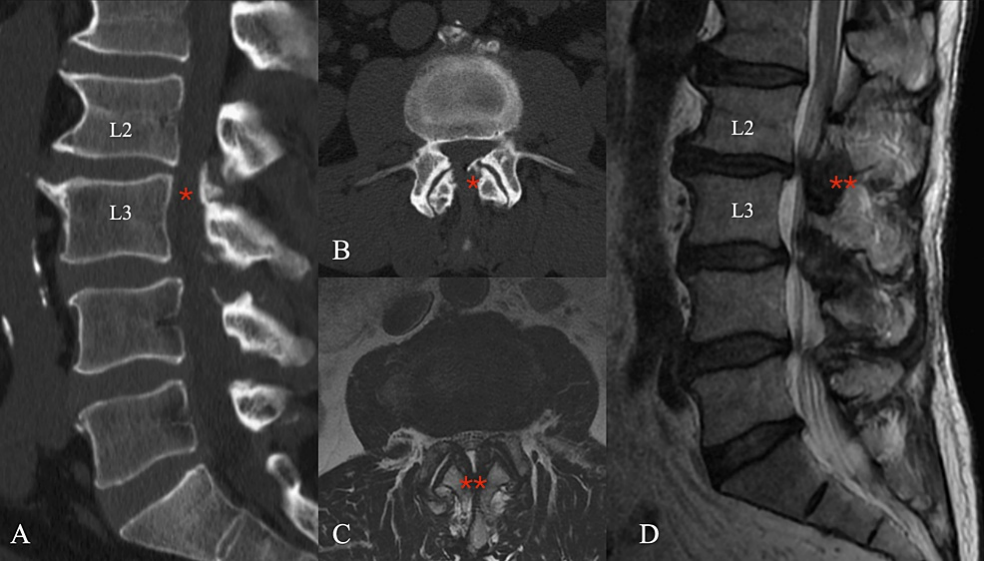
Preoperative imaging (A) Sagittal CT confirmed the protrusion of osteophytes (*) from the superior border of the vertebral arch at the L3 level (B), and osteophytes were confirmed to lie within the spinal canal. (C, D) Axial and sagittal MRI showed that the thickening of the ligamentum flavum () was compressing against the spinal canal.
